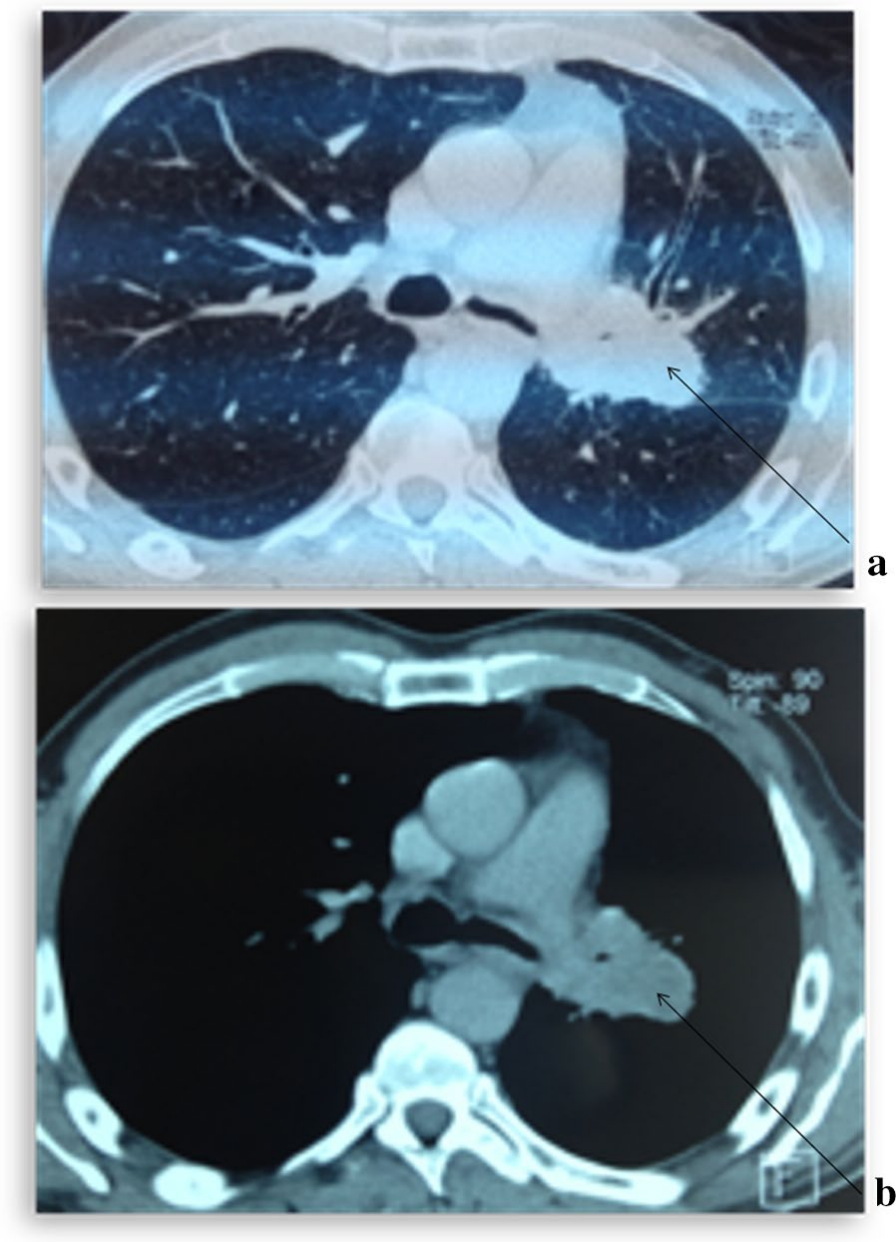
Computed tomography of the chest in pulmonary side (a) and mediastinal side (b). Helical chest CT scan. Axial cut after contrast injection. The pulmonary window (a) showed a heterogeneous tissue process developed at the expense of the posterior segment of the left upper lobe spiculated contours with lymphangitis around the tumor. This tumor measuring 5.5 × 6 cm. On mediastinal window (b): there were no pathologic mediastinal lymph nodes. Vascular axes were free and had normal caliber
